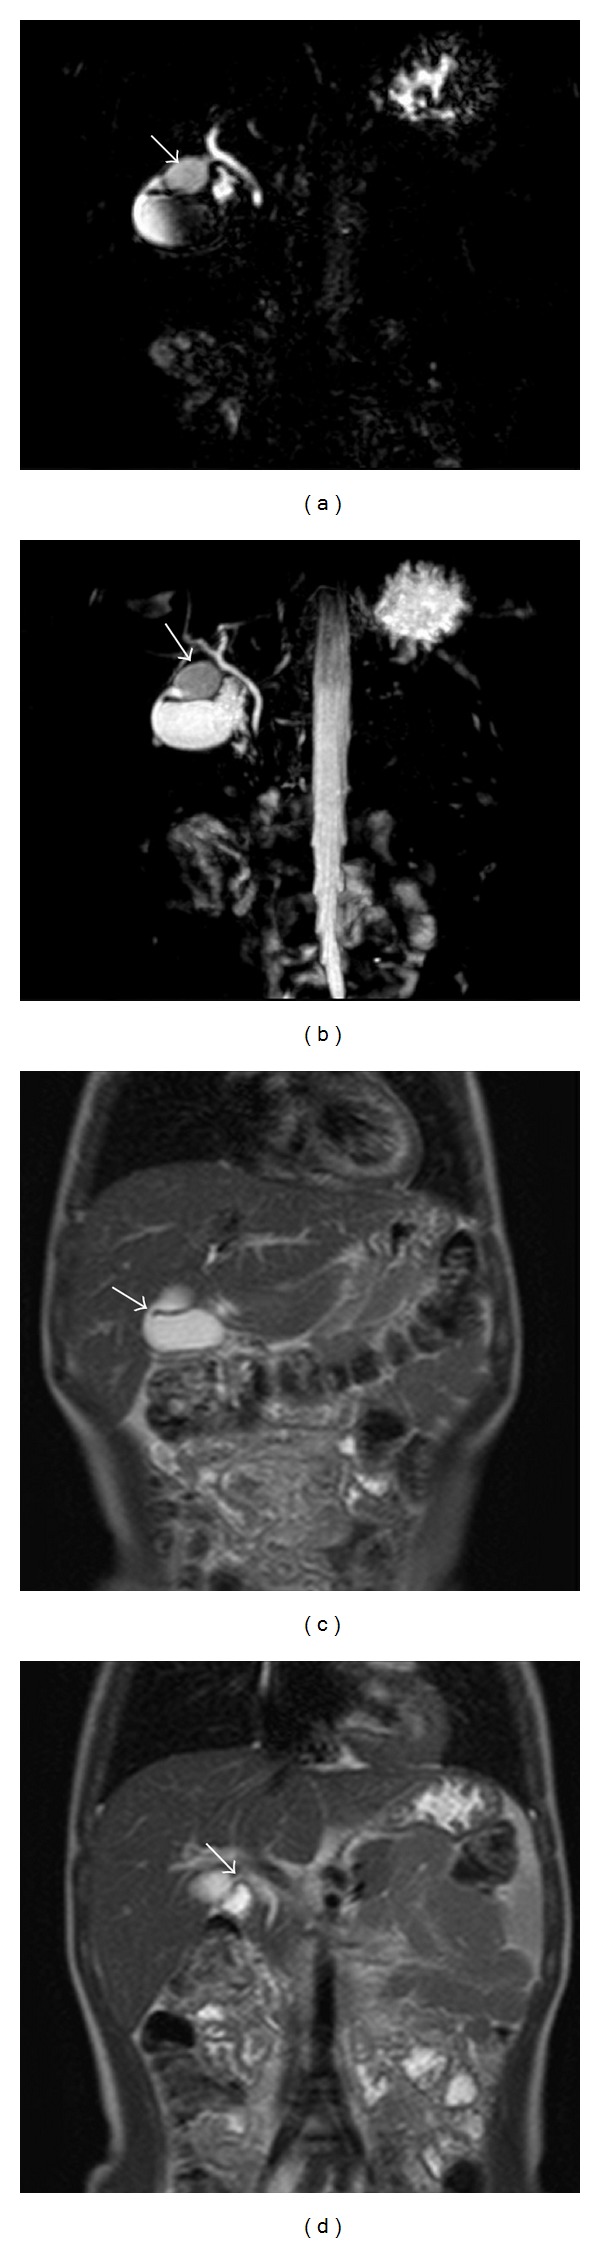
Cystic dilatation of the cystic duct was determined in coronal MRCP and T2-weighted MR images. ((a), (b)) Cystic dilatation is observed in the cystic duct in MRCP images (arrow). The intrahepatic bile ducts and the choledochus are normal. The connection of the dilated cystic duct segment with both gall bladder (c) and choledochus (d) could be determined (arrows).
